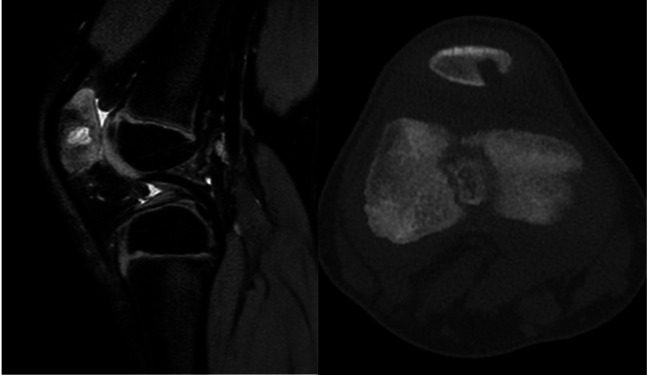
MRI revealed a lytic lesion of the patella in a 4-year-old girl, that was not visible on conventional radiographs. In this case, the condition of the articular cartilage was the key factor that determined the treatment strategy. The lytic lesion was due to *Moraxella lacunata*.
